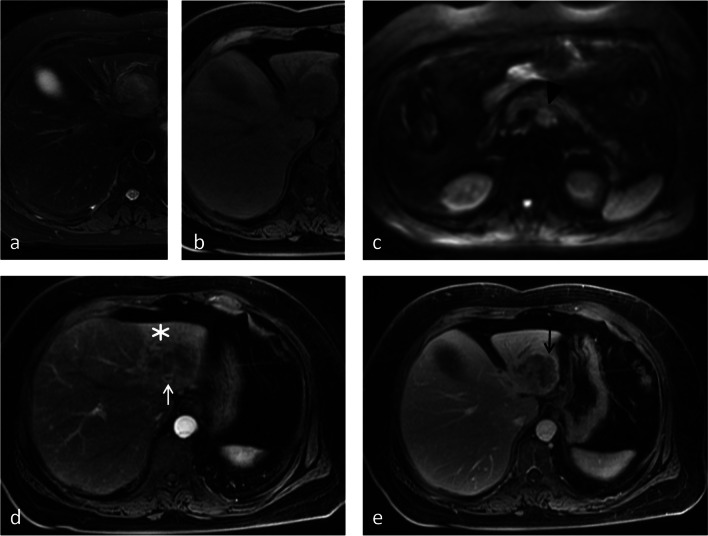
Images from a 78-year-old female with IMCC. a The T2-weighted image and (b) T1-weighted image show a 4.5-cm lesion in segment II of the liver without hepatic capsular retraction. c The DWI image shows an enlarged retroperitoneal lymph node (black arrowhead). d The arterial phase image shows peripheral hepatic enhancement (star) and vessel penetrating the tumor (white arrow). e The portal venous phase image shows rim enhancement (black arrow). Thus, a score of 2 was assigned for this patient
